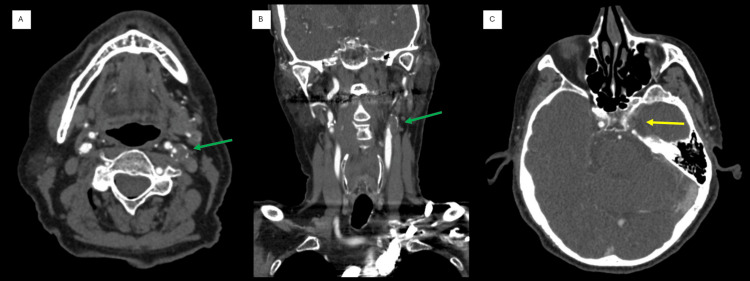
CT angiogram of the head and neck. CT angiogram of the neck showing the left internal carotid artery (ICA) occlusion just at the origin (green arrows) in the axial (A) and coronal (B) views. Axial CT angiogram of the head (C) showing the occlusion extending to the cavernous section of the left ICA (yellow arrow). CT: computed tomography
